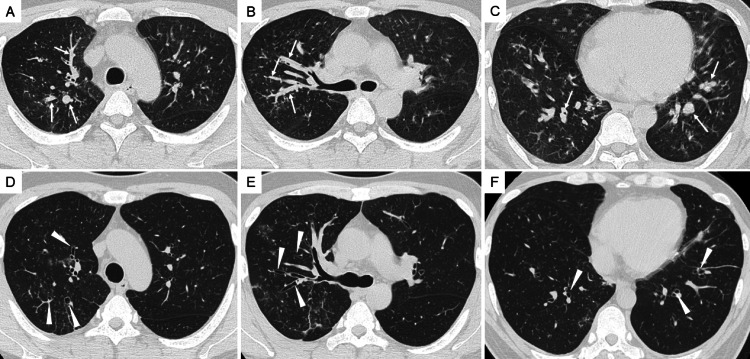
Chest CT before and after bronchoscopic mucus plug removal. (A-C) Chest CT on admission demonstrates impacted mucus plugs (arrows). Arrows in (A) and (B) indicate thick, high-attenuation mucus obstructing the right upper lobe bronchus, while the arrow in (C) highlights mucus impaction within the bilateral lower lobe bronchi, accompanied by central bronchiectasis. (D-F) Follow-up CT performed seven days after bronchoscopic mucus plug removal shows marked resolution of the previously noted mucus impaction (arrowheads) and restoration of airway patency in all affected bronchi.
